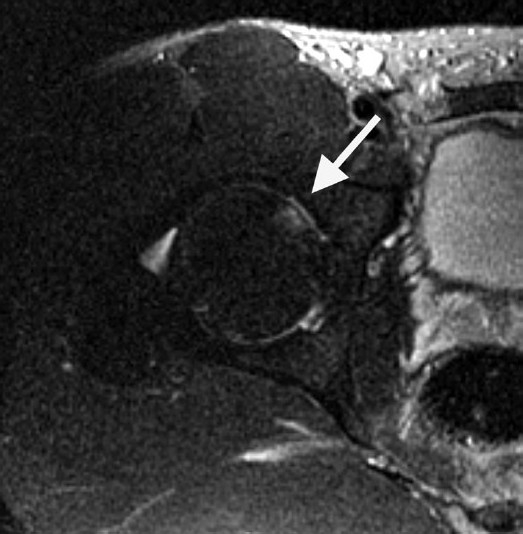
MRI 10 months after injury was normal except for a tiny focus of residual edema in the anterior aspect of the right femoral head
